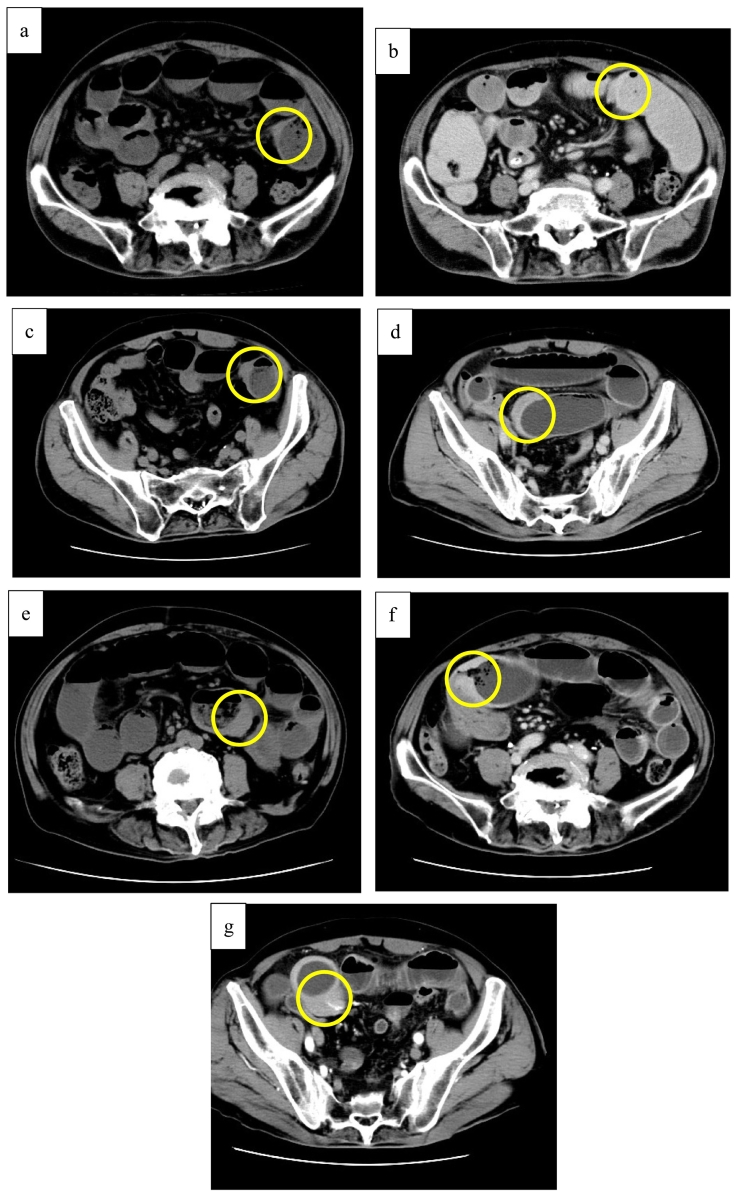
CT images. Retrospective evaluation of the tumor's chronological changes. Tumor locations are indicated by circles. (a) 2021 (b), (c) 2022, (d), (e) 2023, (f), (g) 2024.
